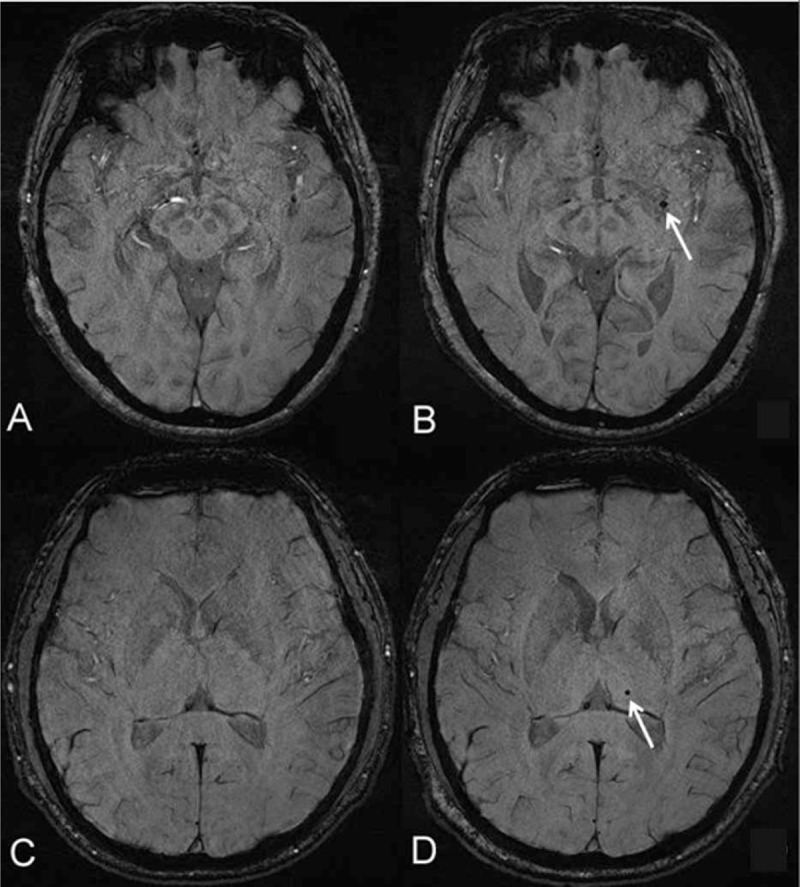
Temporal changes of cerebral microbleeds (CMBs) in patients with hemodialysis over a follow-up MRI. (A, B) A 64-year-old female patient with an interval 17 months of dialysis duration from the first MRI examination. There was a newly developed CMB (white arrow, B) in the left basal ganglia compared with the baseline examination at the same level (A). (C, D) A 30-year-old male patient with an interval 22 months of dialysis duration from the initial MRI examination. There was a newly developed CMB (white arrow, D) in the left dorsal thalamus compared with the baseline examination at the same level (C).
